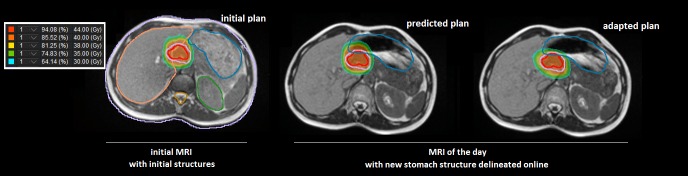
Contours and dose distributions for initial, predicted and adapted treatment plans for the first fraction. Stomach (Blue), gross tumor volume (red) and planning target volume (purple) are displayed on all plans. Position and filling of the stomach on the daily image were very different from initial plan. The predicted plan shows the dose distribution of the initial plan calculated on the current anatomy with updated contours, resulting in excessive dose to the stomach. The adapted plan was re-optimized to avoid excessive dose to the stomach. Isodoses color-lines are displayed in the left box. MRI: Magnetic resonance imaging; Gy: Gray
